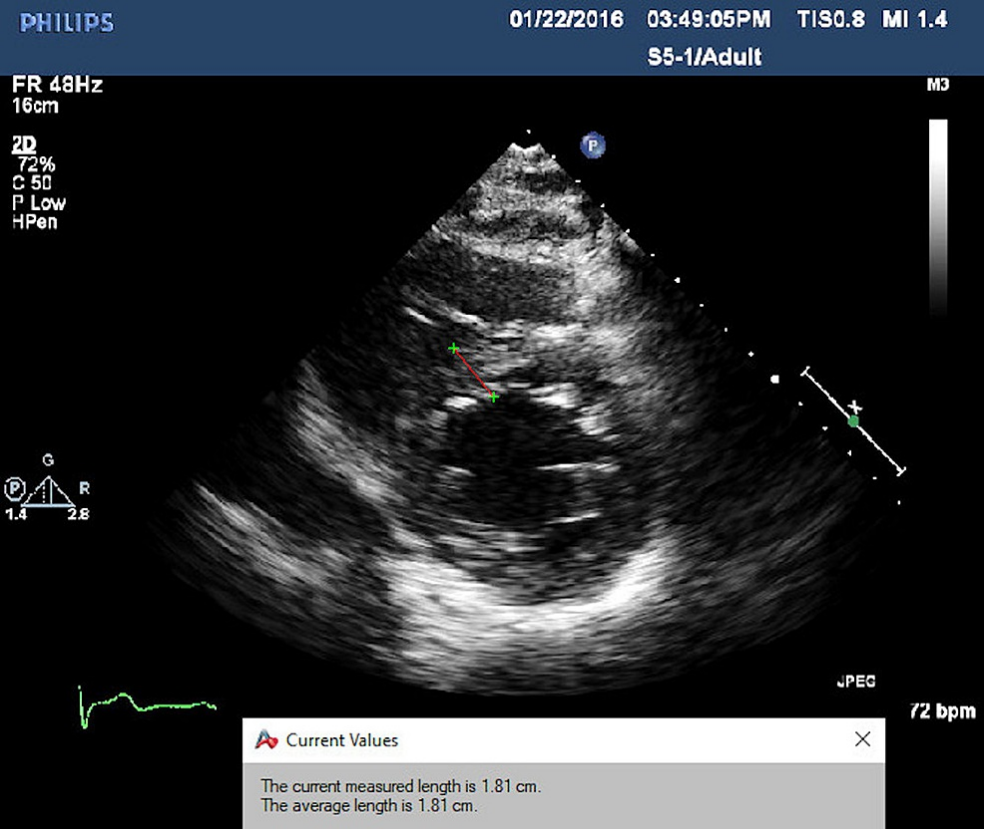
The parasternal short axis shows the concentric ventricular hypertrophy
